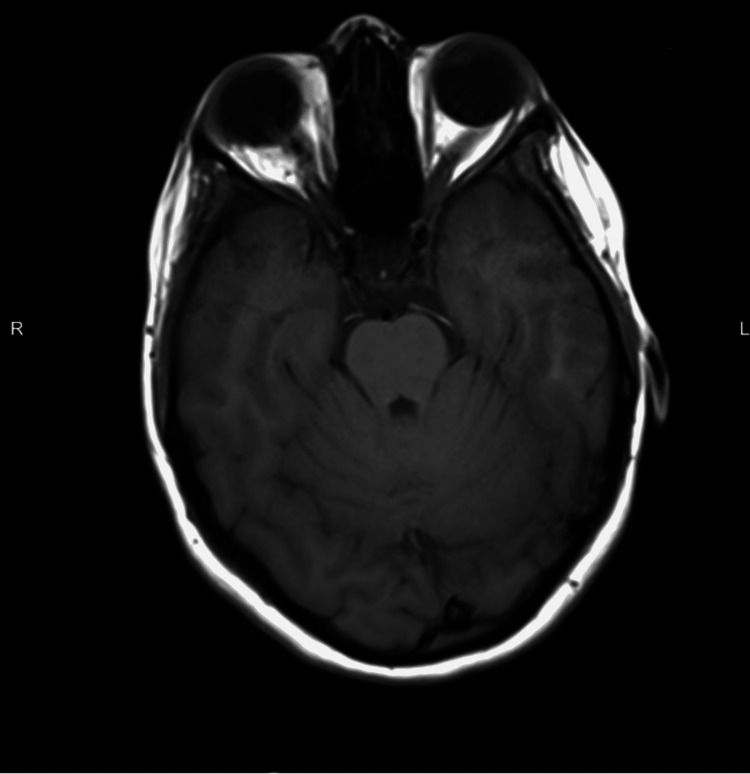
Axial section of brain MRI showing leptomeningeal enhancement
